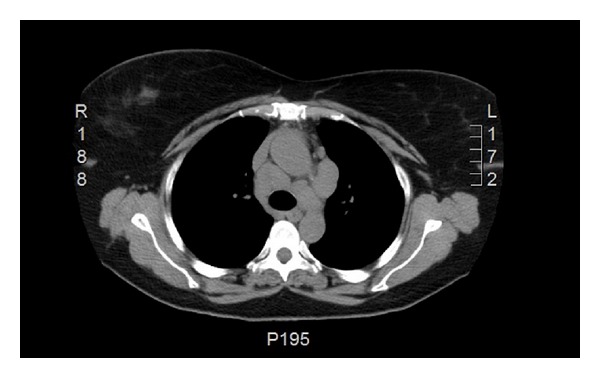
HRCT of the thorax demonstrating mediastinal lymph nodes and the largest was 2.3 × 3.1 centimeters.
